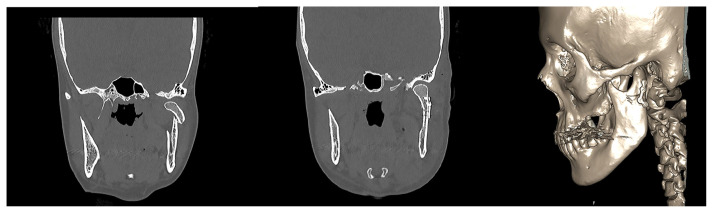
Postoperative CT scan of a condyle fracture after fixation.
